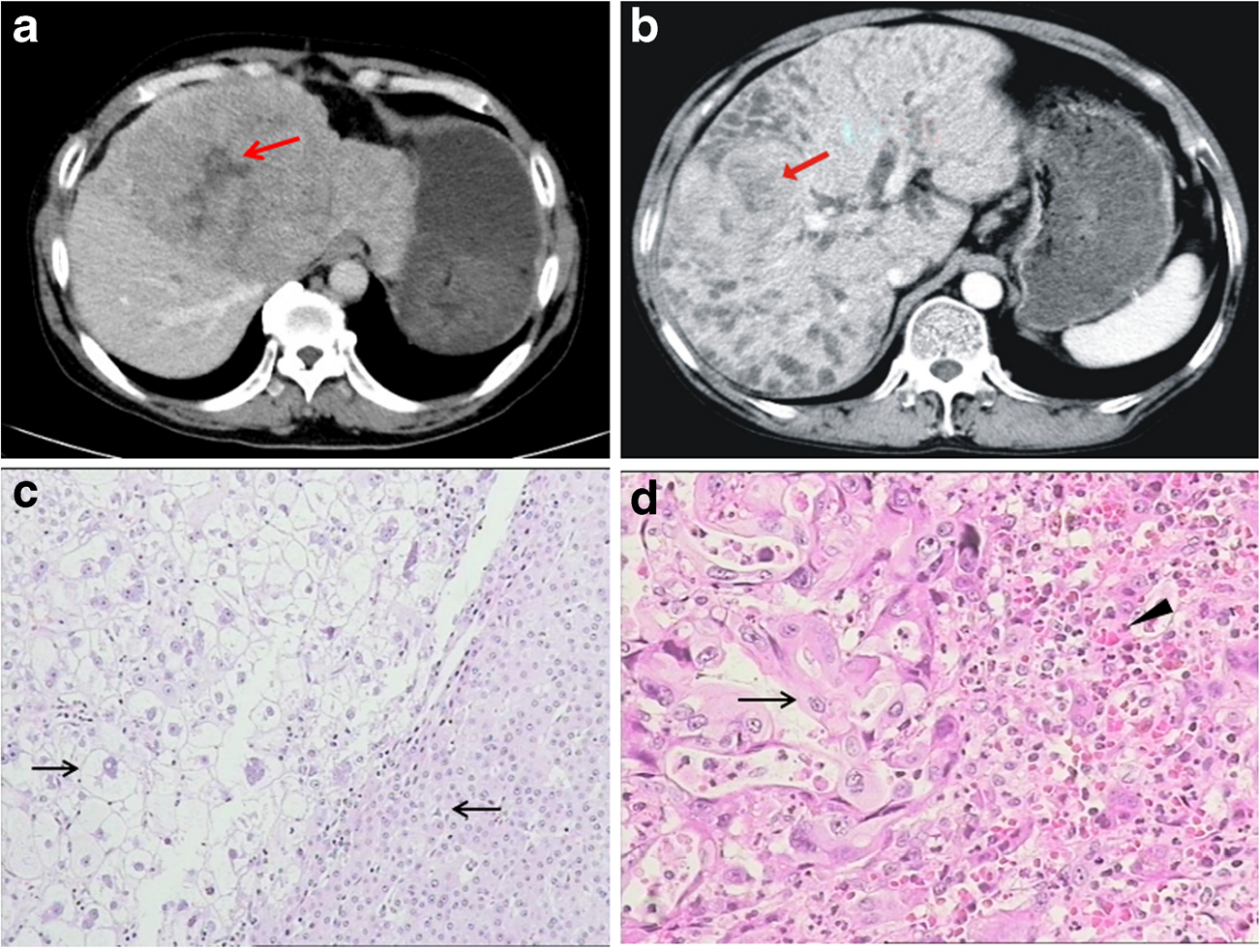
CT diagnosis and biopsy of cholangiocarcinoma and hepatocellular carcinoma. a CT diagnosis of hepatocellular carcinoma. b CT diagnosis of intrahepatic cholangiocarcinoma. c Liver biopsy of hepatocellular carcinoma (magnification ×400). d Liver biopsy of cholangiocarcinoma (magnification ×400)
